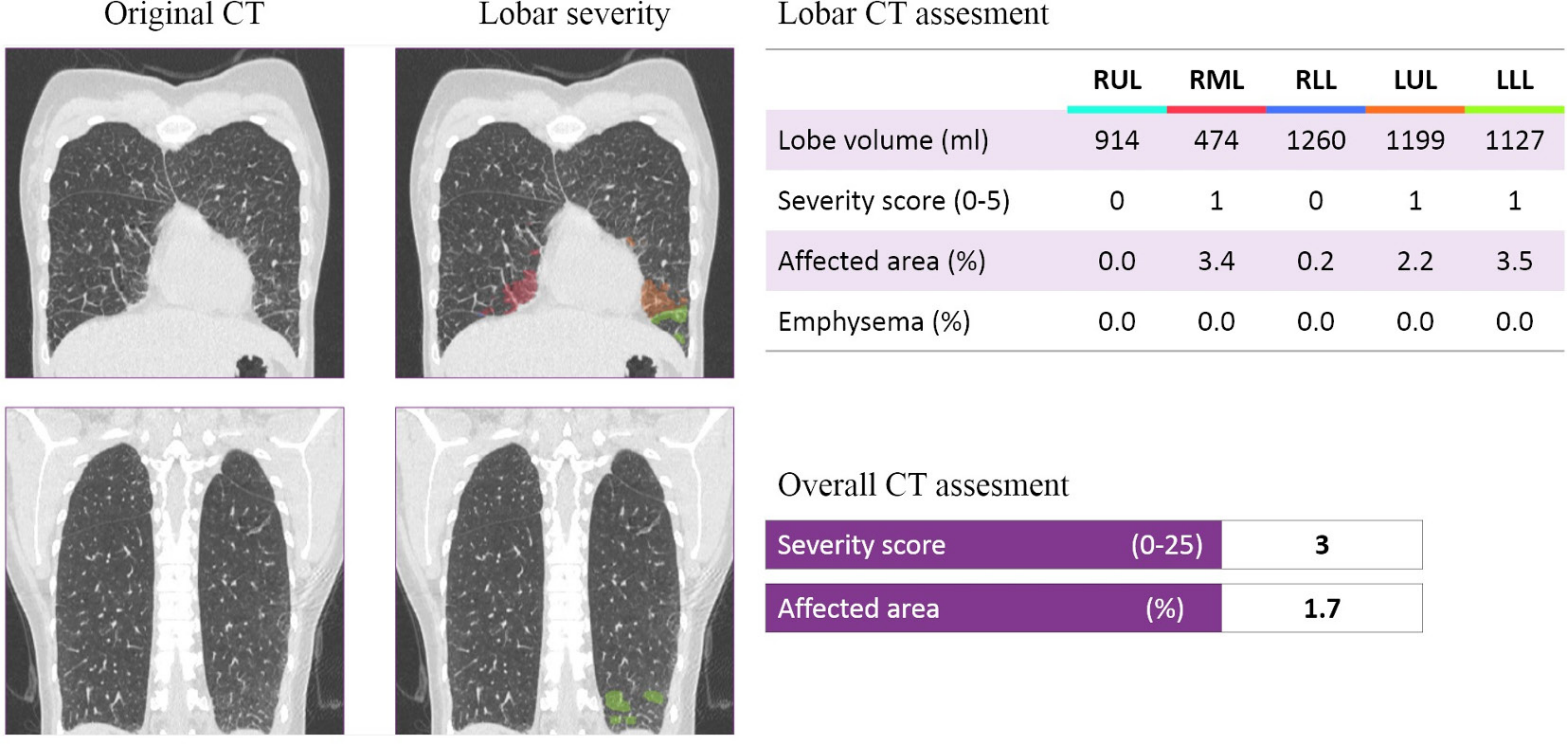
Representative example of a COVID-19 patient with a severity score of 3. The original and DL-assessed chest CT of a 41-year-old female patient, who tested negative for COVID-19.
